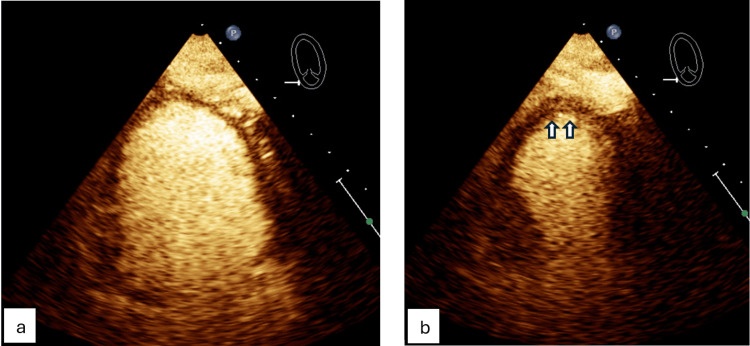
Prior transthoracic echocardiogram (apical four-chamber view) showing apical ballooning of the left ventricle. (a) Wall motion in diastole. (b) Wall motion in systole.
